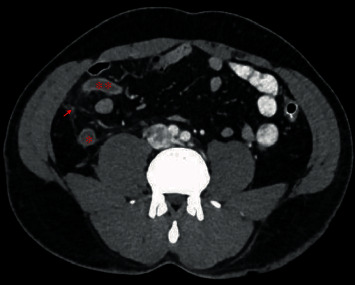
Abdomen CT scan with IV and oral contrast showing dilated appendicular tip (^∗∗^) and base (^∗^), with periappendiceal fat stranding (arrow) and enhancing wall.
